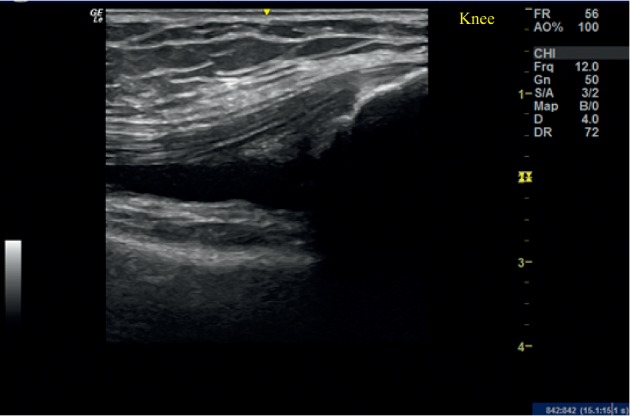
Ultrasound examination of the right knee in case 3 at 8 weeks. Longitudinal view showing synovial effusion 8 weeks after PRP injection.
